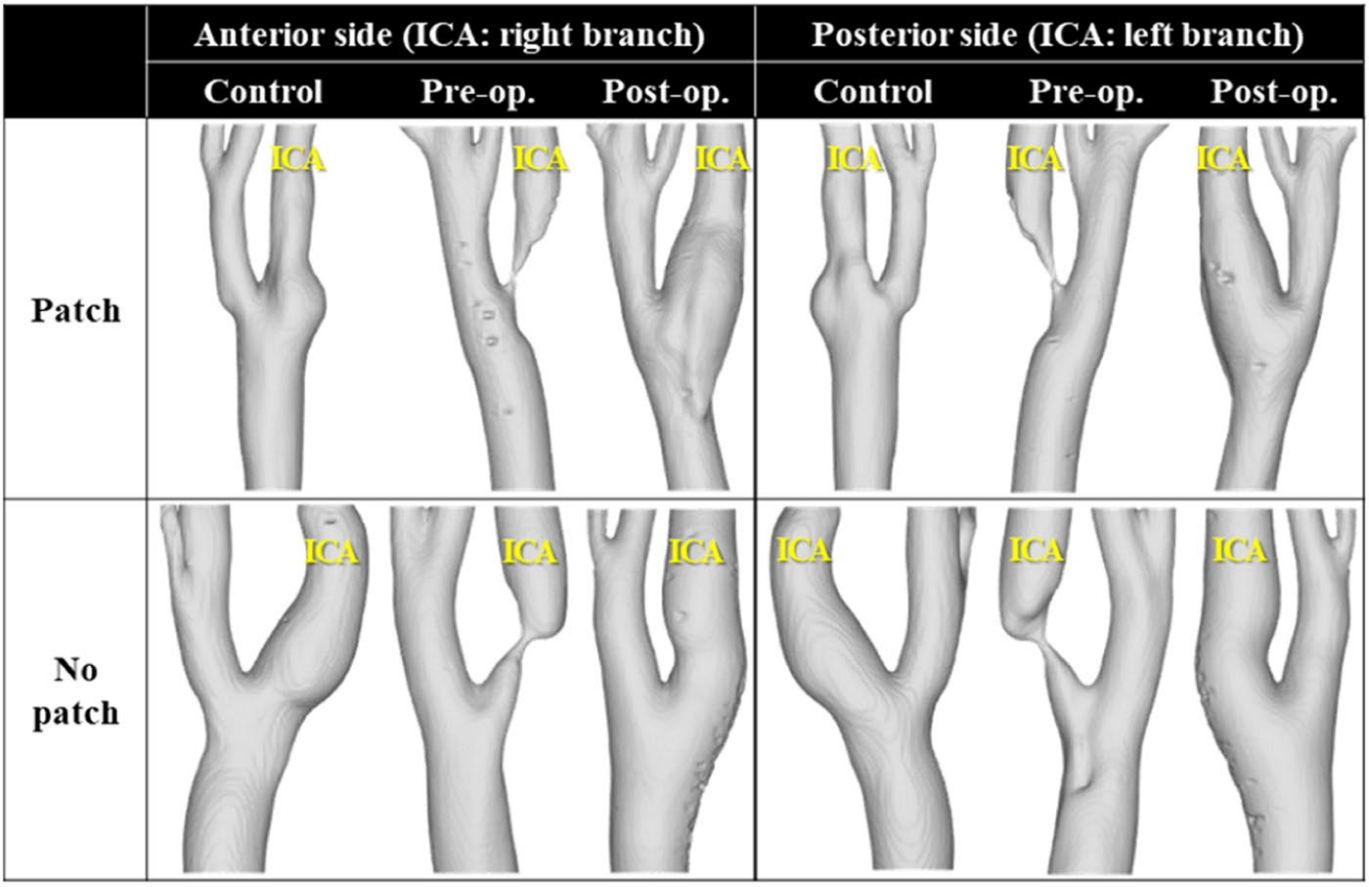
Reconstructed carotid geometries from 4D flow MRI measurements. The contralateral carotid was selected as the control for each patient.
